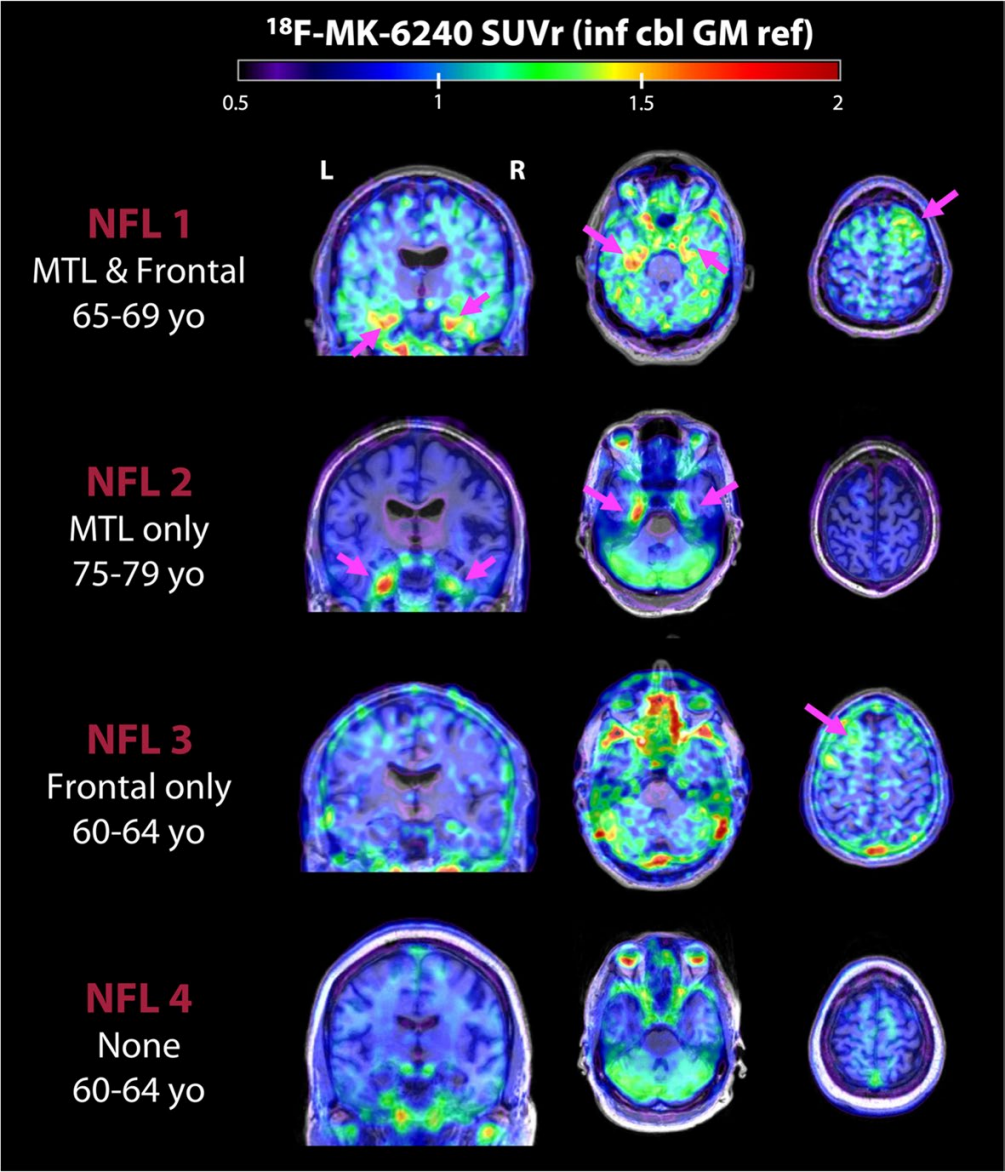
Example ^18^F-MK-6240 scans in four former NFL players. Each row shows data from a single participant. Image slices depict 70-90 min SUVr, referenced against inferior cerebellar gray matter and overlaid on native space MRIs. Pink arrows point to areas with elevated cortical signal according to visual read
